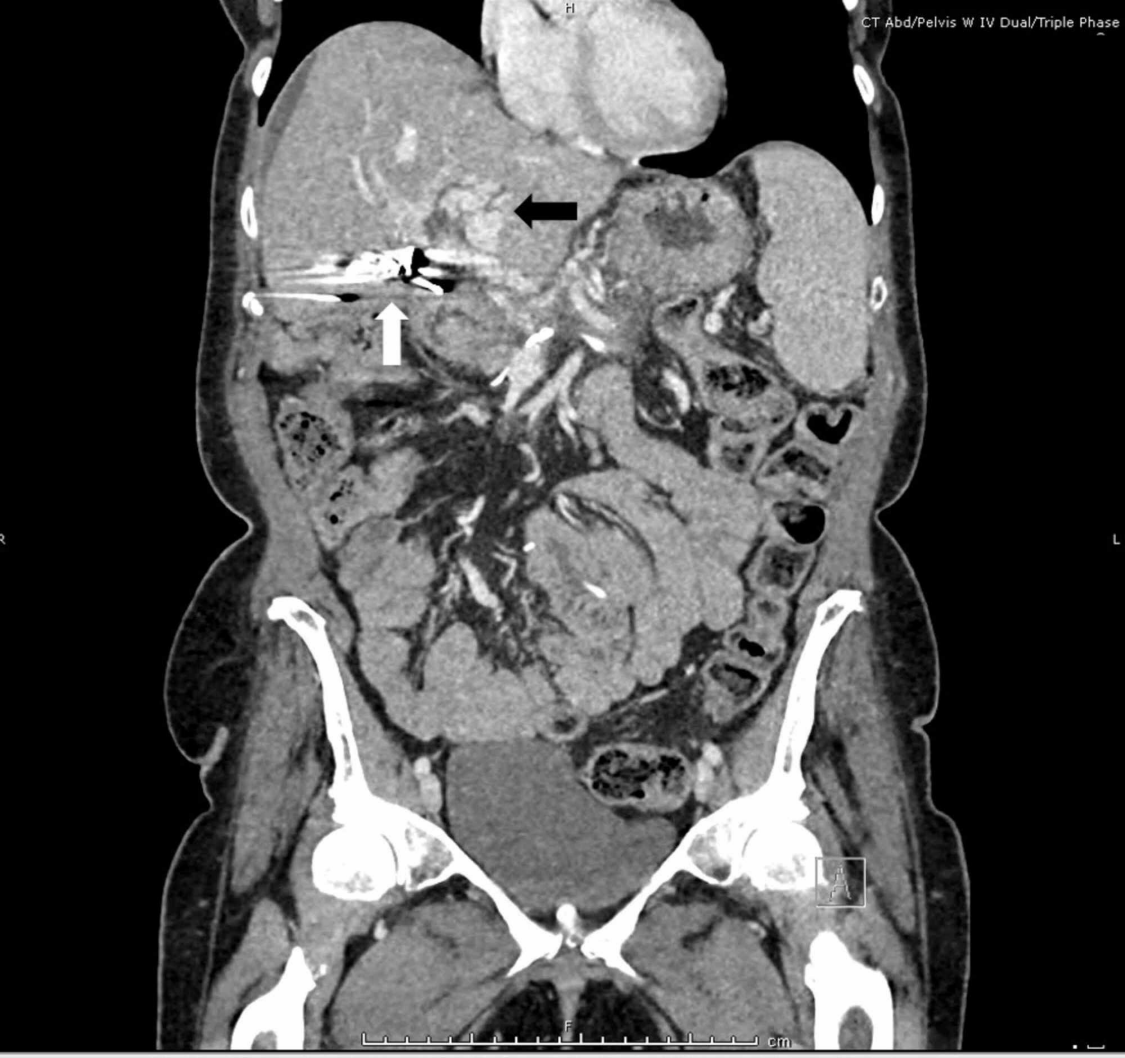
Computed tomography angiography of the abdomen and pelvis shows cavernous transformation (black arrow) and metallic artifact from previous surgery (white arrow) with no active bleeding
